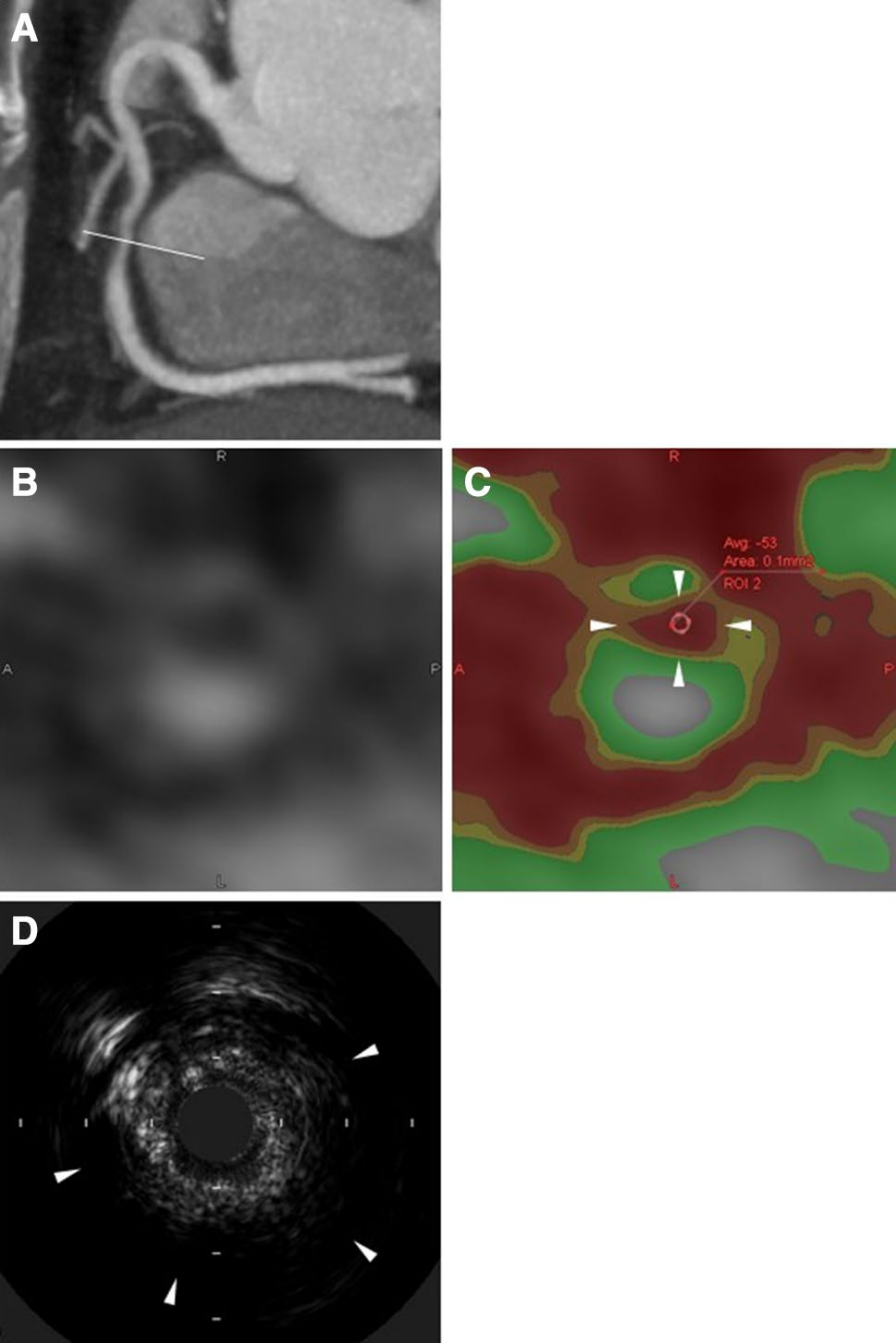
Representative images of CTA v-LAP (a–c) and IVUS AP (d). a Longitudinal coronary image and cross-sectional point by slab maximum intensity projection image. b Grayscale cross-sectional image. c Color map image and measurement of minimum CT density. Red area indicates < 0 HU density, i.e., v-LAP (arrowheads), orange area ≥ 0 and < 30 HU, yellow area ≥ 30 and < 50 HU, and green area ≥ 50 and < 250 HU. d Arrowheads indicate attenuated plaque on IVUS. *CTA* computed tomography angiography, *HU* Hounsfield units, *IVUS* intravascular ultrasound, *IVUS AP* attenuated plaque on IVUS, *v*-*LAP* very low attenuation plaque
